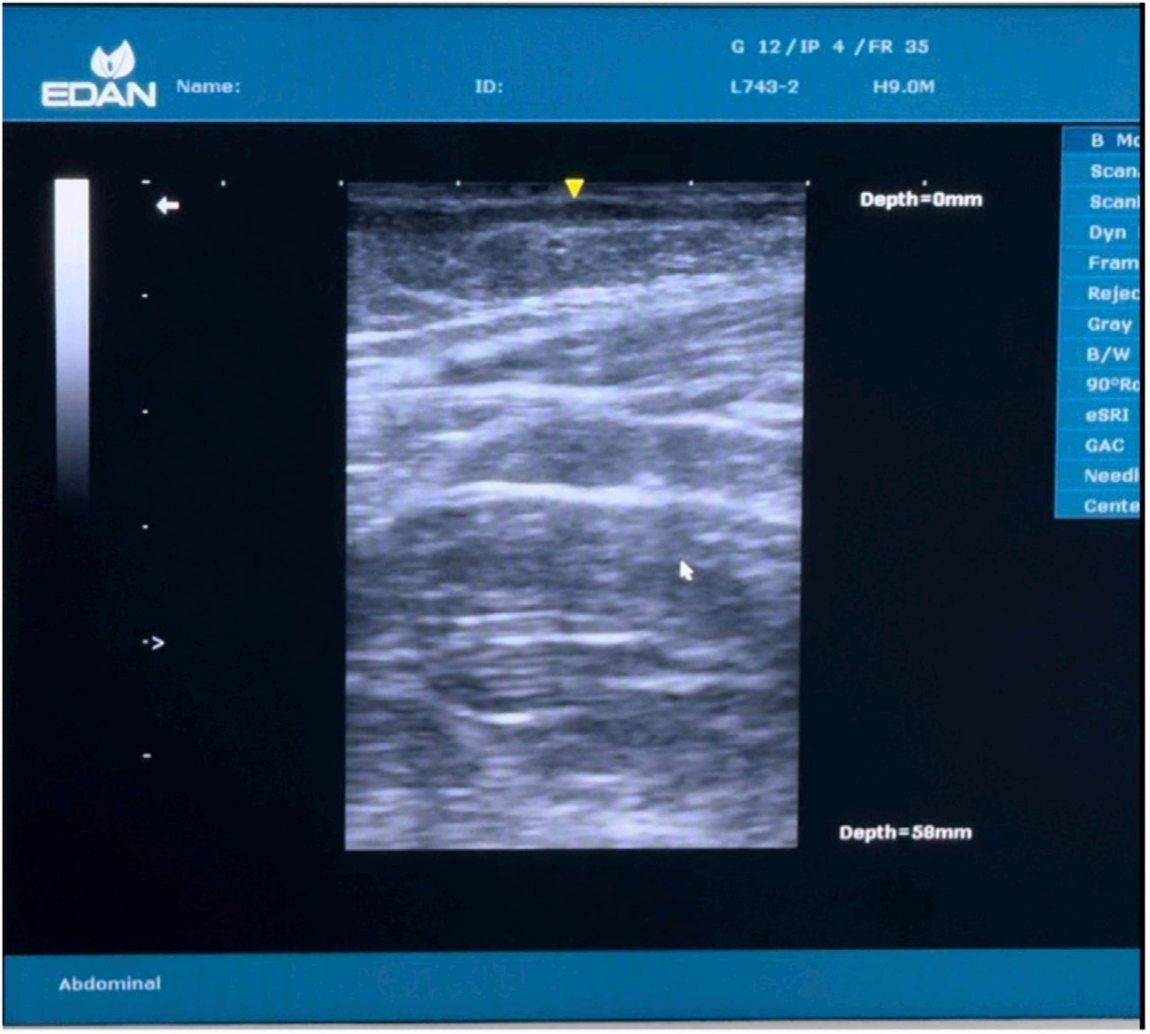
Ultrasound image showing 3 layers.
